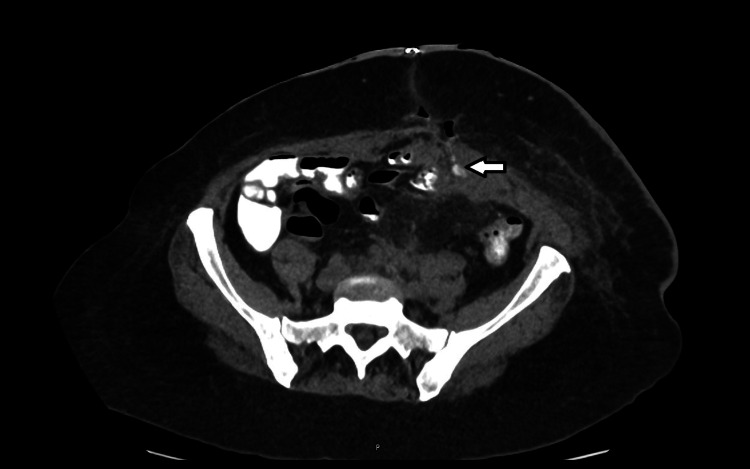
Axial view with an enterocutaneous fistula (white arrow) Repeat CT scan showing the fistula
